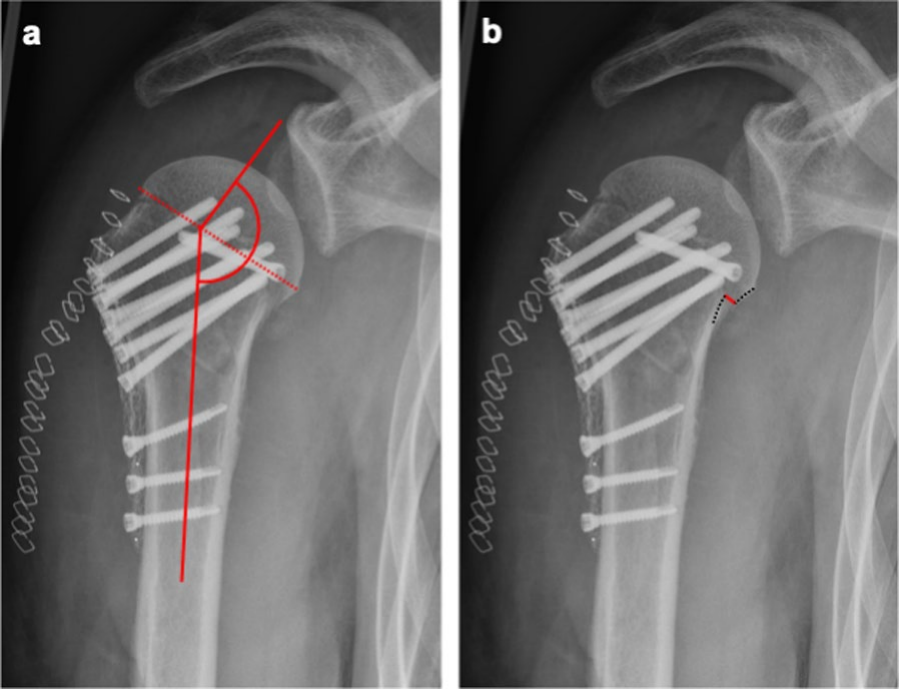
Radiological measurements in an anterior posterior radiograph: a neck shaft angulation, b neck shaft distance
